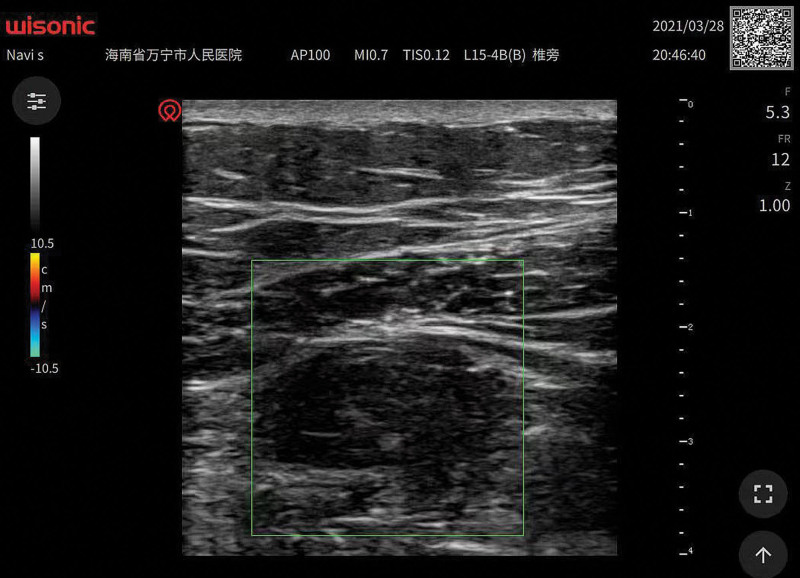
High-frequency probe QLB and TAP block path in supine position: SF = subcutaneous fat, LD = latissimus dorsi muscle, ES = erector muscle, SPI = serratus posterior inferior muscle, EO = external abdominal oblique muscle, IO = internal abdominal oblique muscle, TA = transversal abdominis muscle, TAP = transversus abdominis plane block, QL = quadratus lumborum, QLB = quadratus lumborum block, PM = psoas major muscle, AC = abdominal cavity, N1 = puncture path of quadratus lumborum, N2 = puncture path of transversal abdominal muscle, Green square = Doppler window.
